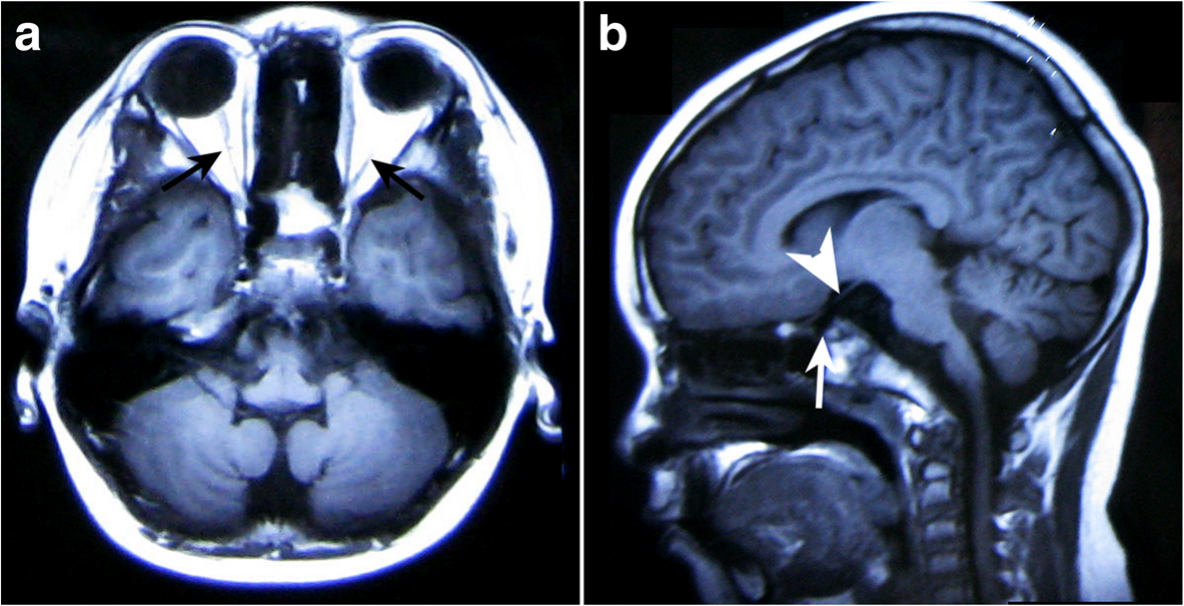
The magnetic resonance imaging (MRI) of the patient with *CISD2* mutation. a: axial T1WI shows bilateral atrophy of optic nerves (black arrows) and (b): sagittal T1WI shows absence of the physiological high signal of the posterior lobe of the pituitary gland (white arrow). The arrow head shows the thinning optic nerves
